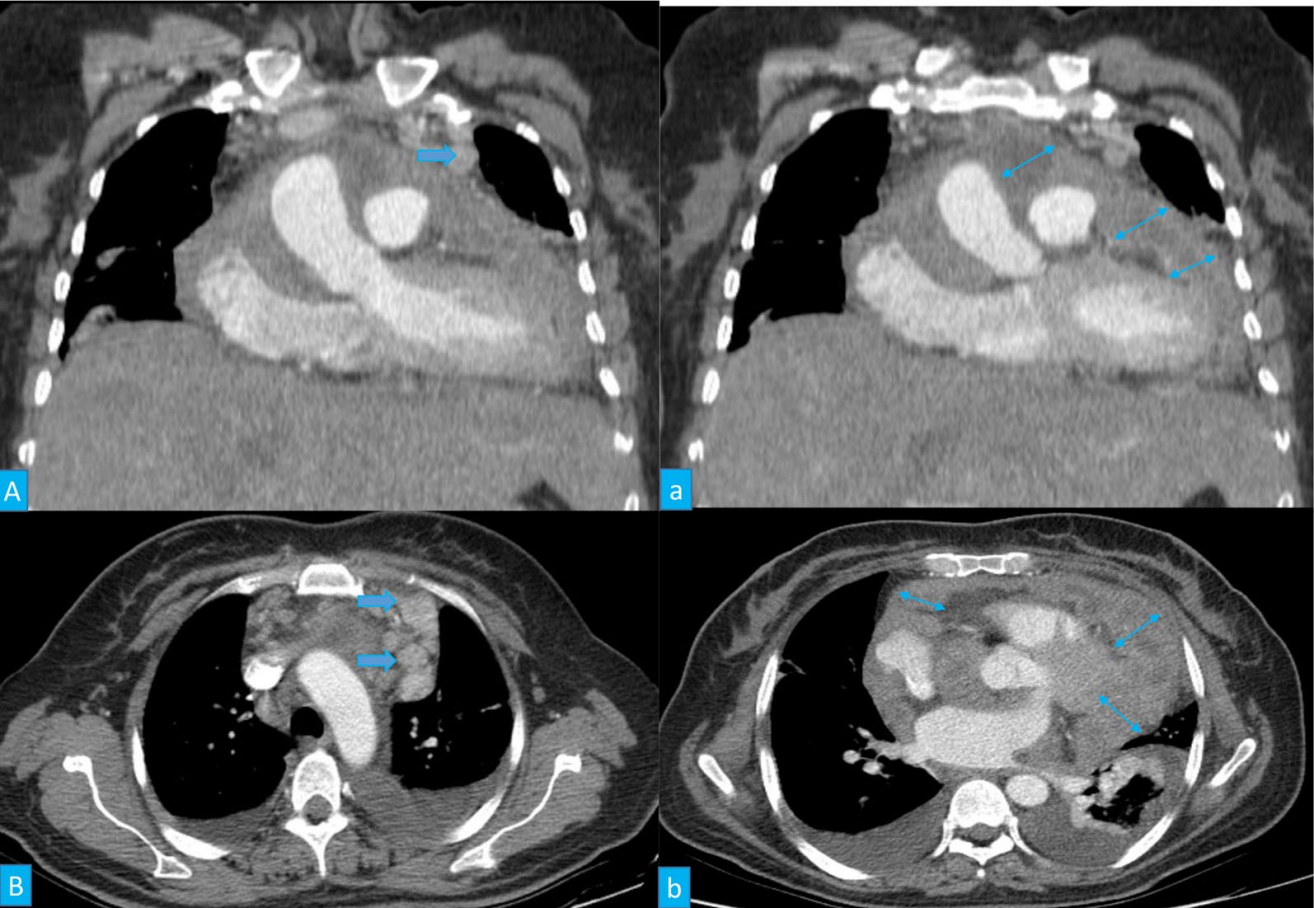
Two reconstructed coronal computed tomography images (A, a) and thin section axial computed tomography images (B, b) revealed some large enhancing nodes in the left anterior mediastinum (arrows), and nodular thickening of the pericardium (double arrows) with irregularity and enhancement associated with effusion compressing heart chambers
